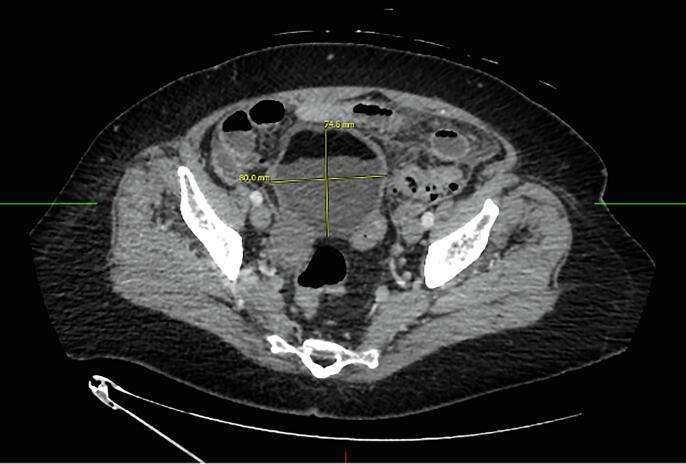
CT AP with contrast when patient initially presented to the hospital. 8.0 cm thick walled mass containing fat, fluid, soft tissue and calcification arising from the right ovary.
